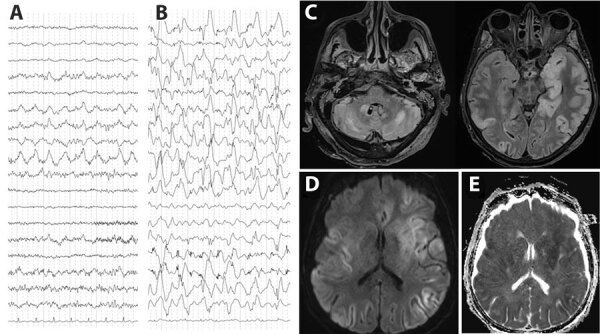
Electroencephalogram (EEG) and brain magnetic resonance imaging (MRI) testing of a patient with encephalitis caused by pigeon paramyxovirus type 1, France. A) The first EEG (day 5 after hospitalization) revealed nonspecific encephalopathy consisting of disorganized theta background rhythm and right temporal rhythmic delta focalization without seizure. B) A later EEG (day 23 after hospitalization) confirmed severe encephalitis with continuous, diffuse, nonreactive, high-amplitude, delta rhythm background of pseudo-periodic delta biphasic and triphasic waves, suggestive of cortical necrosis. C) MRI revealed diffuse bilateral fluid-attenuated inversion recovery hyperintensities, primarily cortical, with subcortical extensions, particularly to the temporal and insular regions, involving the pulvinar nuclei of the thalami, the basal ganglia, the internal capsule, the limbic system, and cerebellum. D, E) Hypersignals in diffusion-weighted images (D) and with reduced apparent diffusion coefficient (E) suggested diffuse cytotoxic brain edema in relation to severe necrotic encephalitis.
